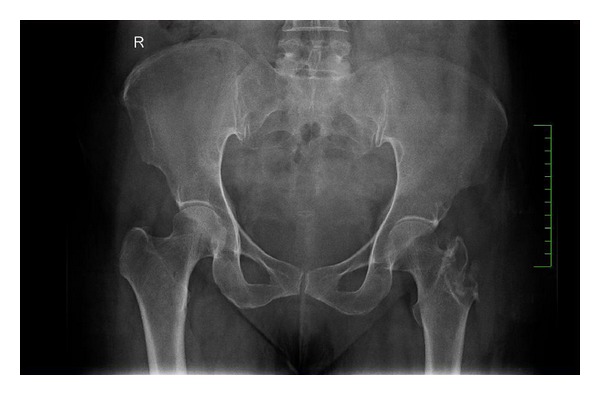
X-ray of the pelvis with both hips showing a well-defined eccentric osteolytic lesion involving the left greater trochanter with an enlarged soft tissue shadow adjacent to it.
